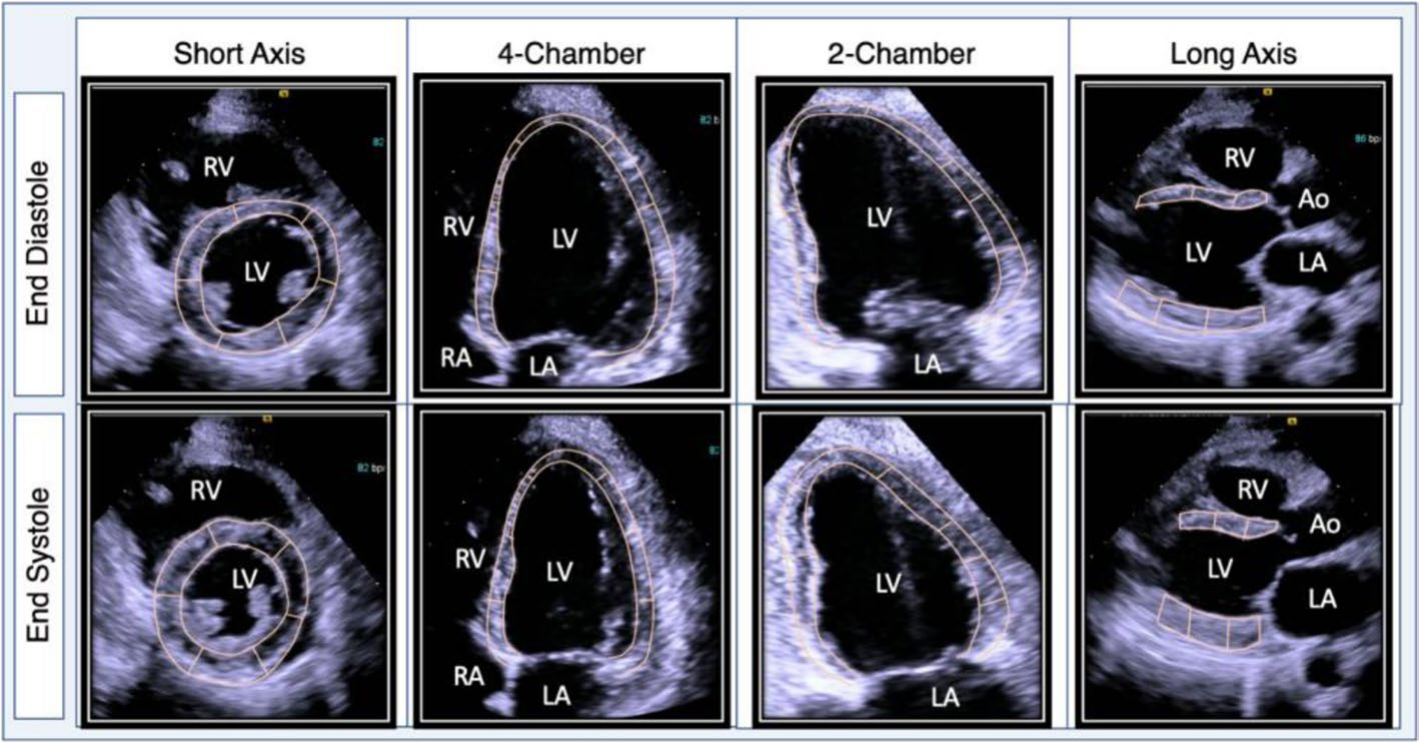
Echocardiogram after four doses of gilteritinib demonstrating acutely reduced systolic function and global hypokinesis. After four doses of gilteritinib targeted therapy, an echocardiogram showed moderately reduced left ventricular systolic function, an estimated ejection fraction of 36% with global hypokinesis and regional abnormalities of the septum
